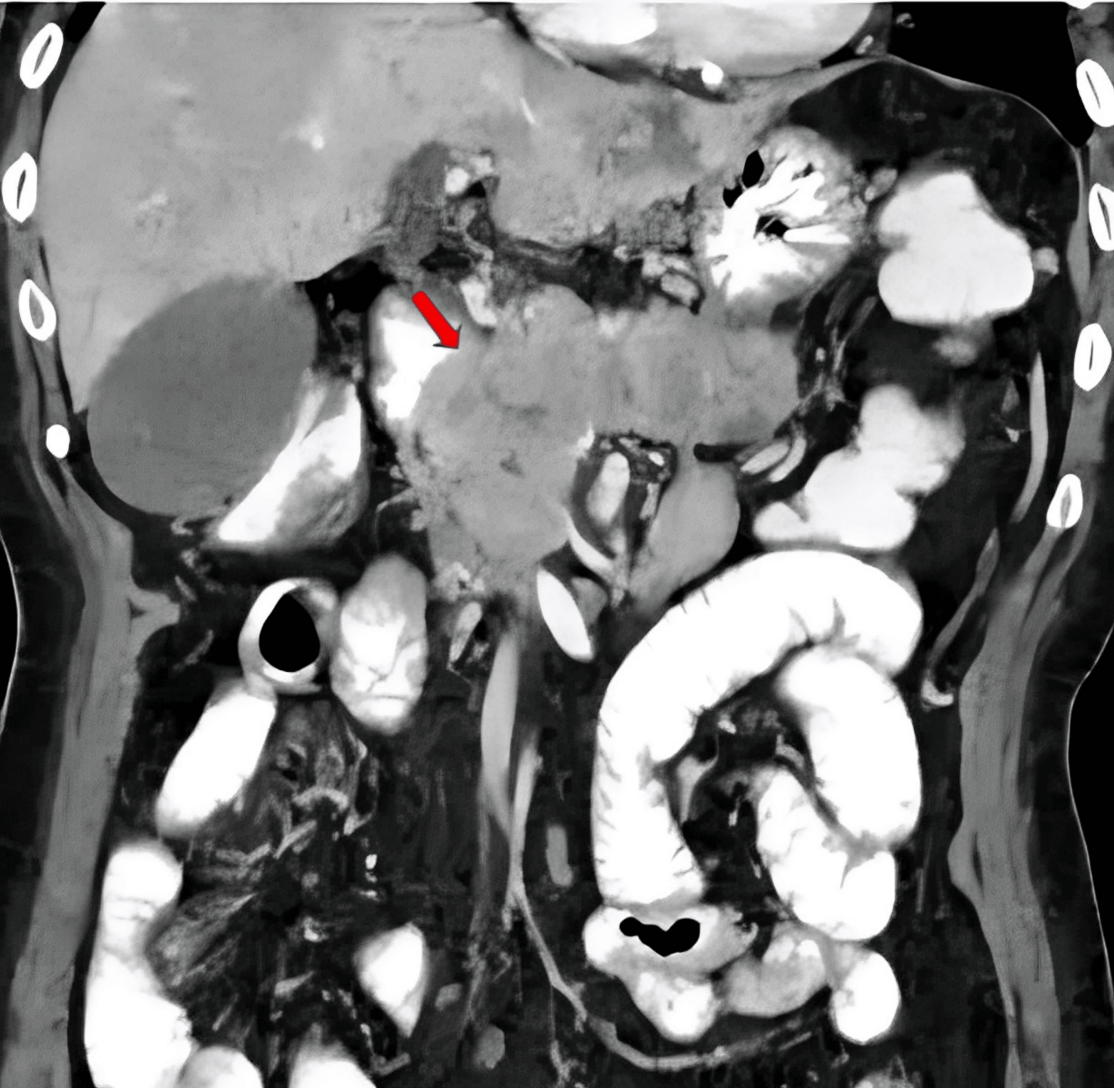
Contrast-enhanced computed tomography of the abdomen showing a mass located in the head of the pancreas (red arrow)
